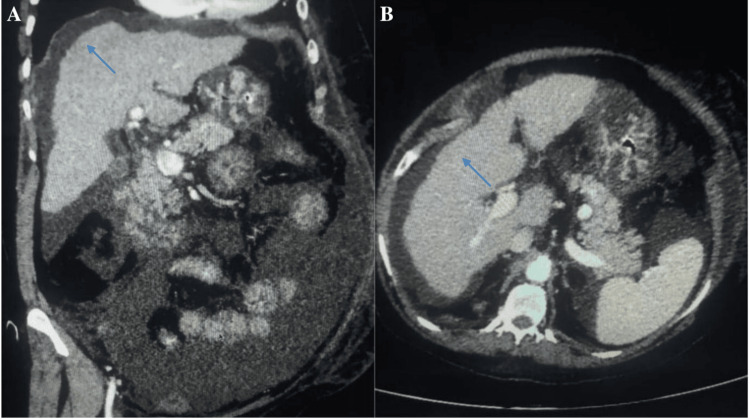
CT of the abdomen transverse (A) and axial (B) sections showing hepatomegaly, heterogeneous liver parenchyma, and features of acute hepatitis (arrows)
